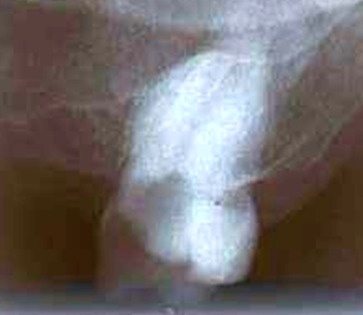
Initial radiograph of maxillary second molar
